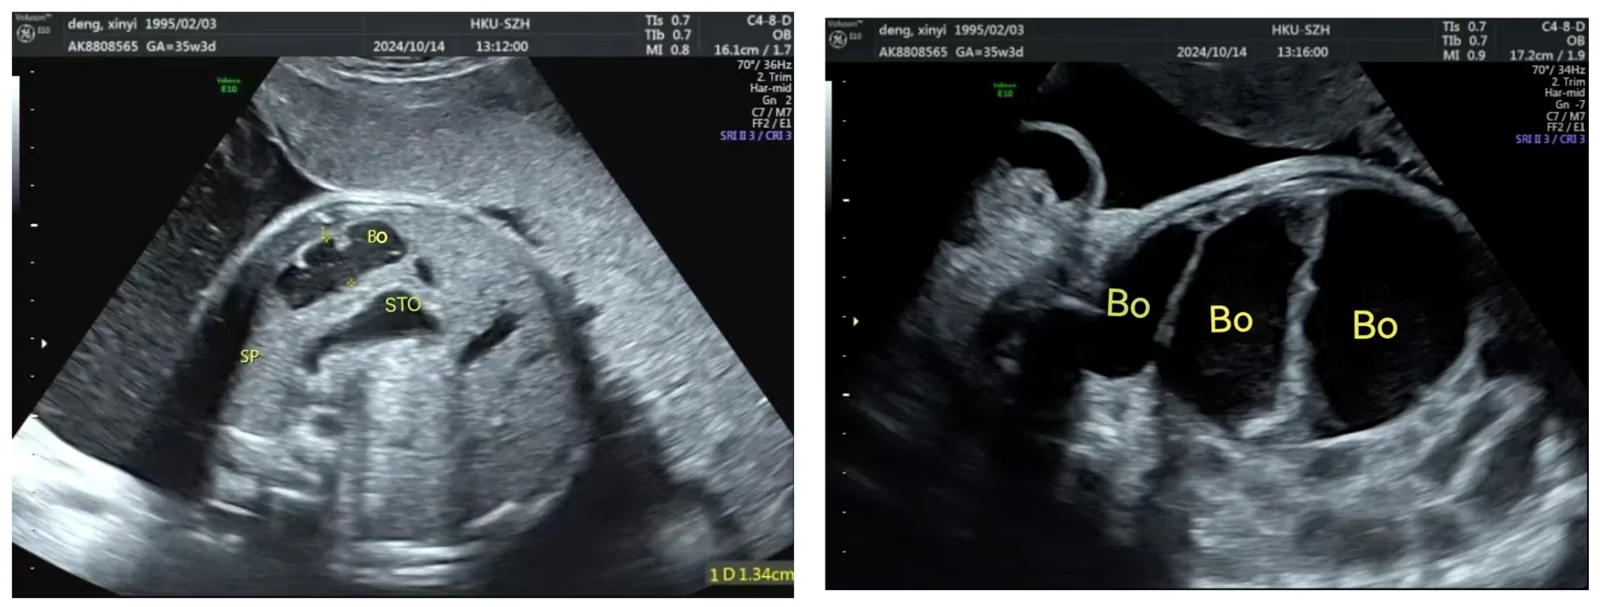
Fetal ultrasound (35 weeks): the fetal bowel appeared dilated in the (left image) and was markedly more dilated in the (right image); a cystic mass-like change resembling a “coffee bean sign” was observed in the fetal abdominal cavity (on retrospective image analysis). STO: stomach; Bo: bowel; SP: spleen. 1: lumen diameter.
